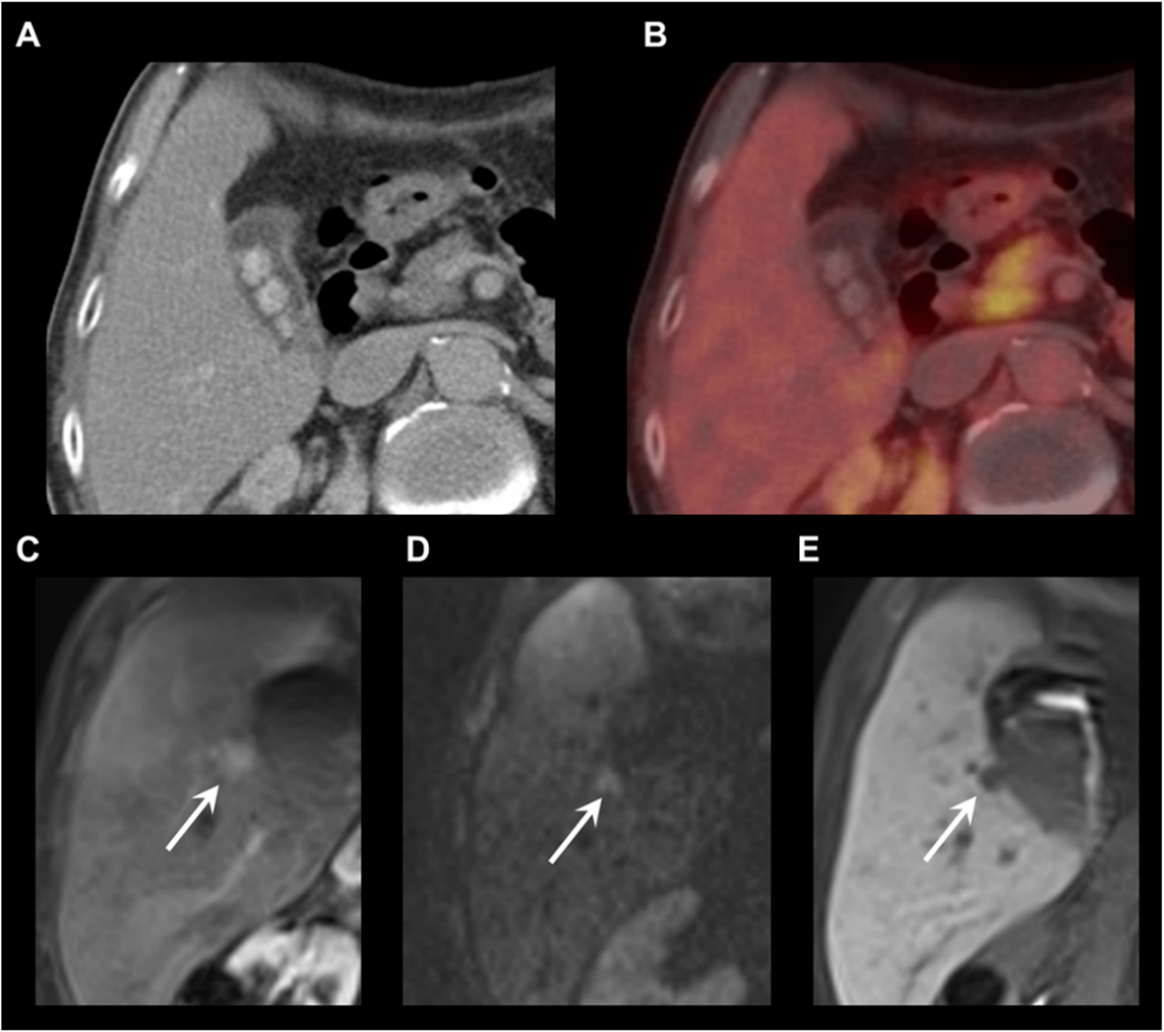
Detection of an ^18^F-DOPA-PET/CT occult liver metastasis on gadoxetic acid-enhanced MRI. a Contrast-enhanced CT. b
